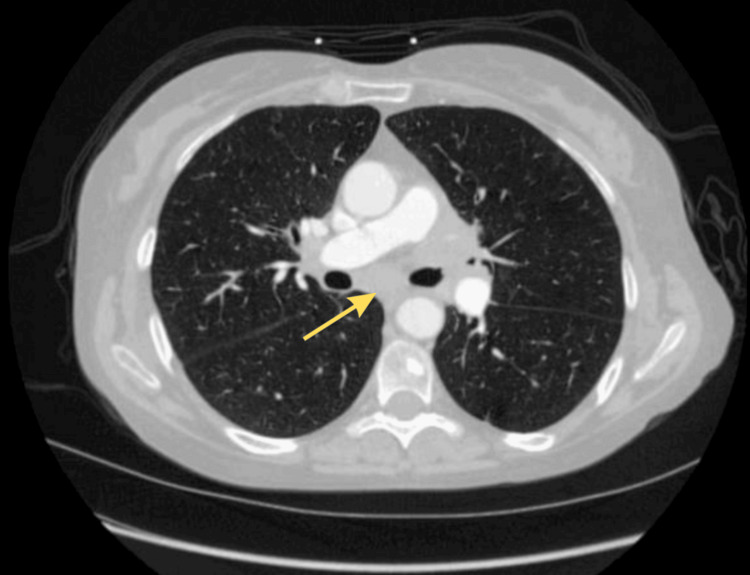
Axial CT scan showing a precarinal mass (yellow arrow) accompanied by multiple enlarged lymph nodes in the aortopulmonary window. CT: Computed tomography
